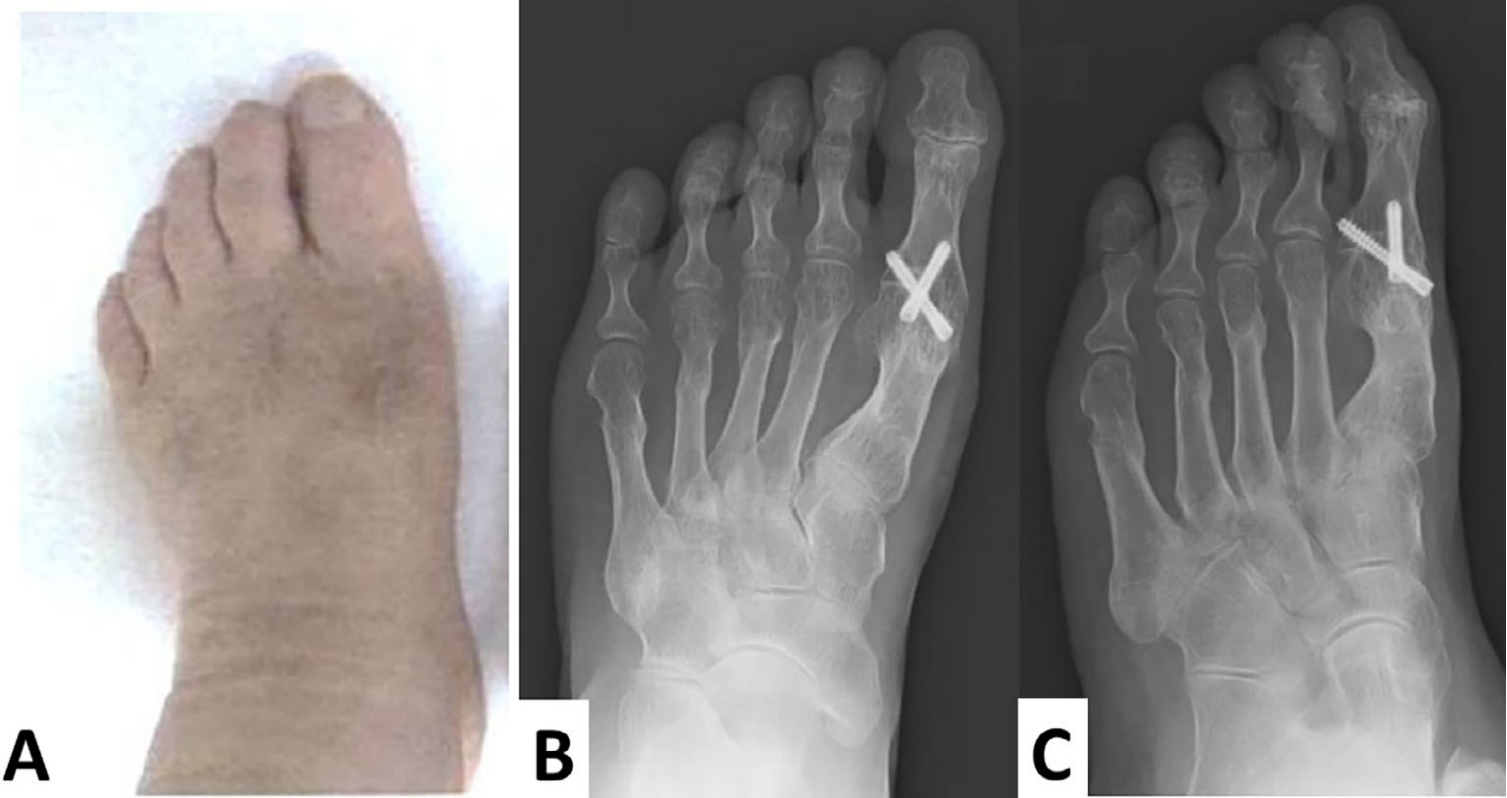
Macroscopic findings and radiographs are taken four years after the operation. No recurrence of hallux varus and hallux valgus is observed (A). The screws are intact, in place, and no valgus or varus deformities are apparent. Additionally, each osteotomized lesser toe is united (B, anteroposterior view; C, oblique view).
